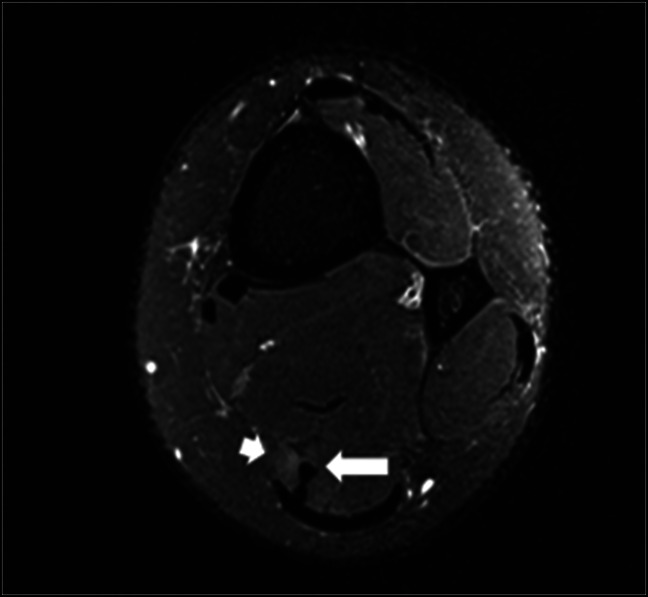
Axial T2FS MR image of the left ankle demonstrating feathery edema within the posterior medial muscle fibers of the Achilles (shorter white arrow) with adjacent anteromedially located low-signal plantaris tendon without tendinopathy (longer white arrow).
